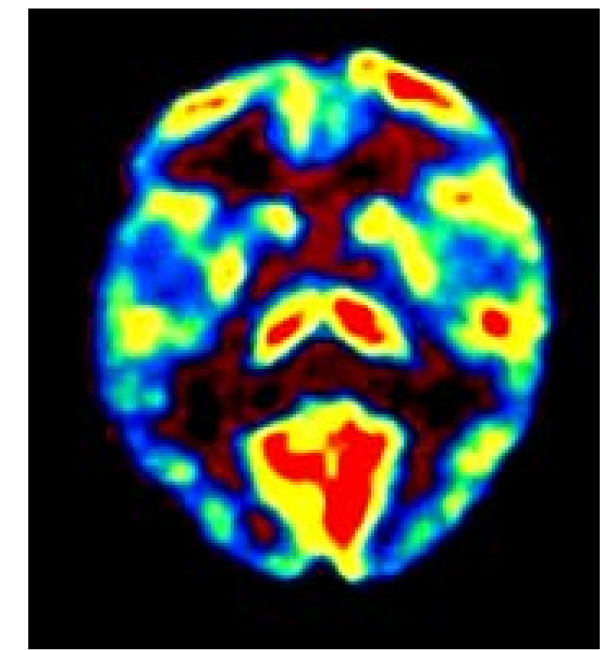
FDG-PET of patient #1. [^18^F] deoxyglucose (FDG) positron emission tomography (PET) had been performed in each patient before treatment. The area of hypermetabolic activity in the temporal cortex was chosen as target for TMS treatment. Here the FDG PET of patient #1 is displayed, where a transversal slice through the temporal brain region shows unilaterally increased metabolic activity in projection to the left auditory cortex.
